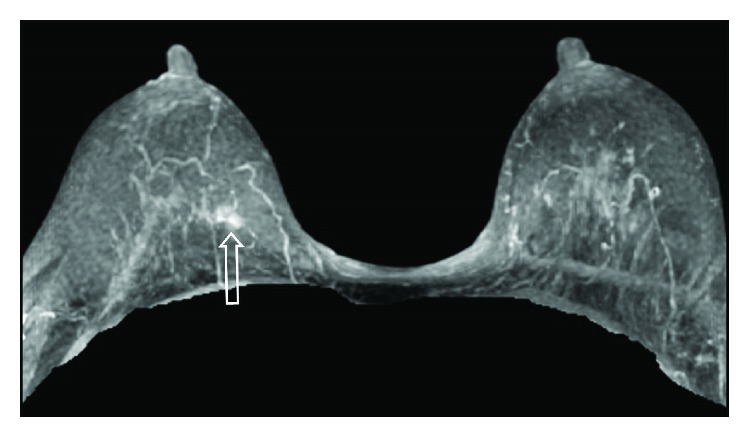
3D maximum intensity projection (MIP) contrast subtraction study MRI of breast on September 15, 2017. There was an about 7 mm focal asymmetry at the posterior inner hemisphere of right breast (arrow).
